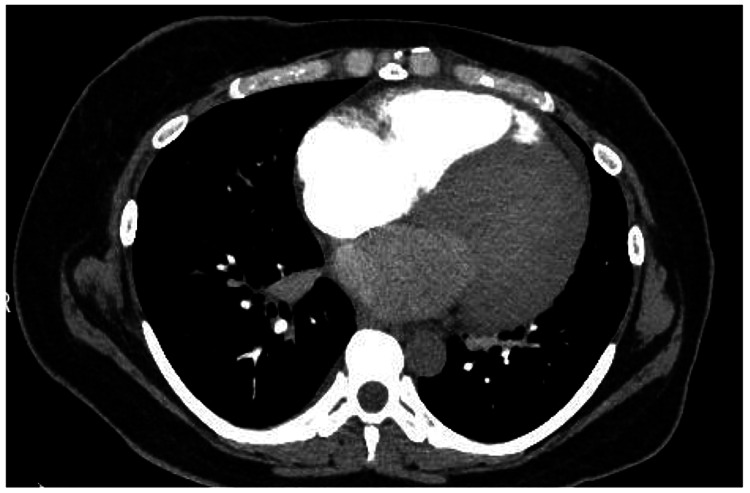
CT of the chest with intravenous contrast showing cardiomegaly.
